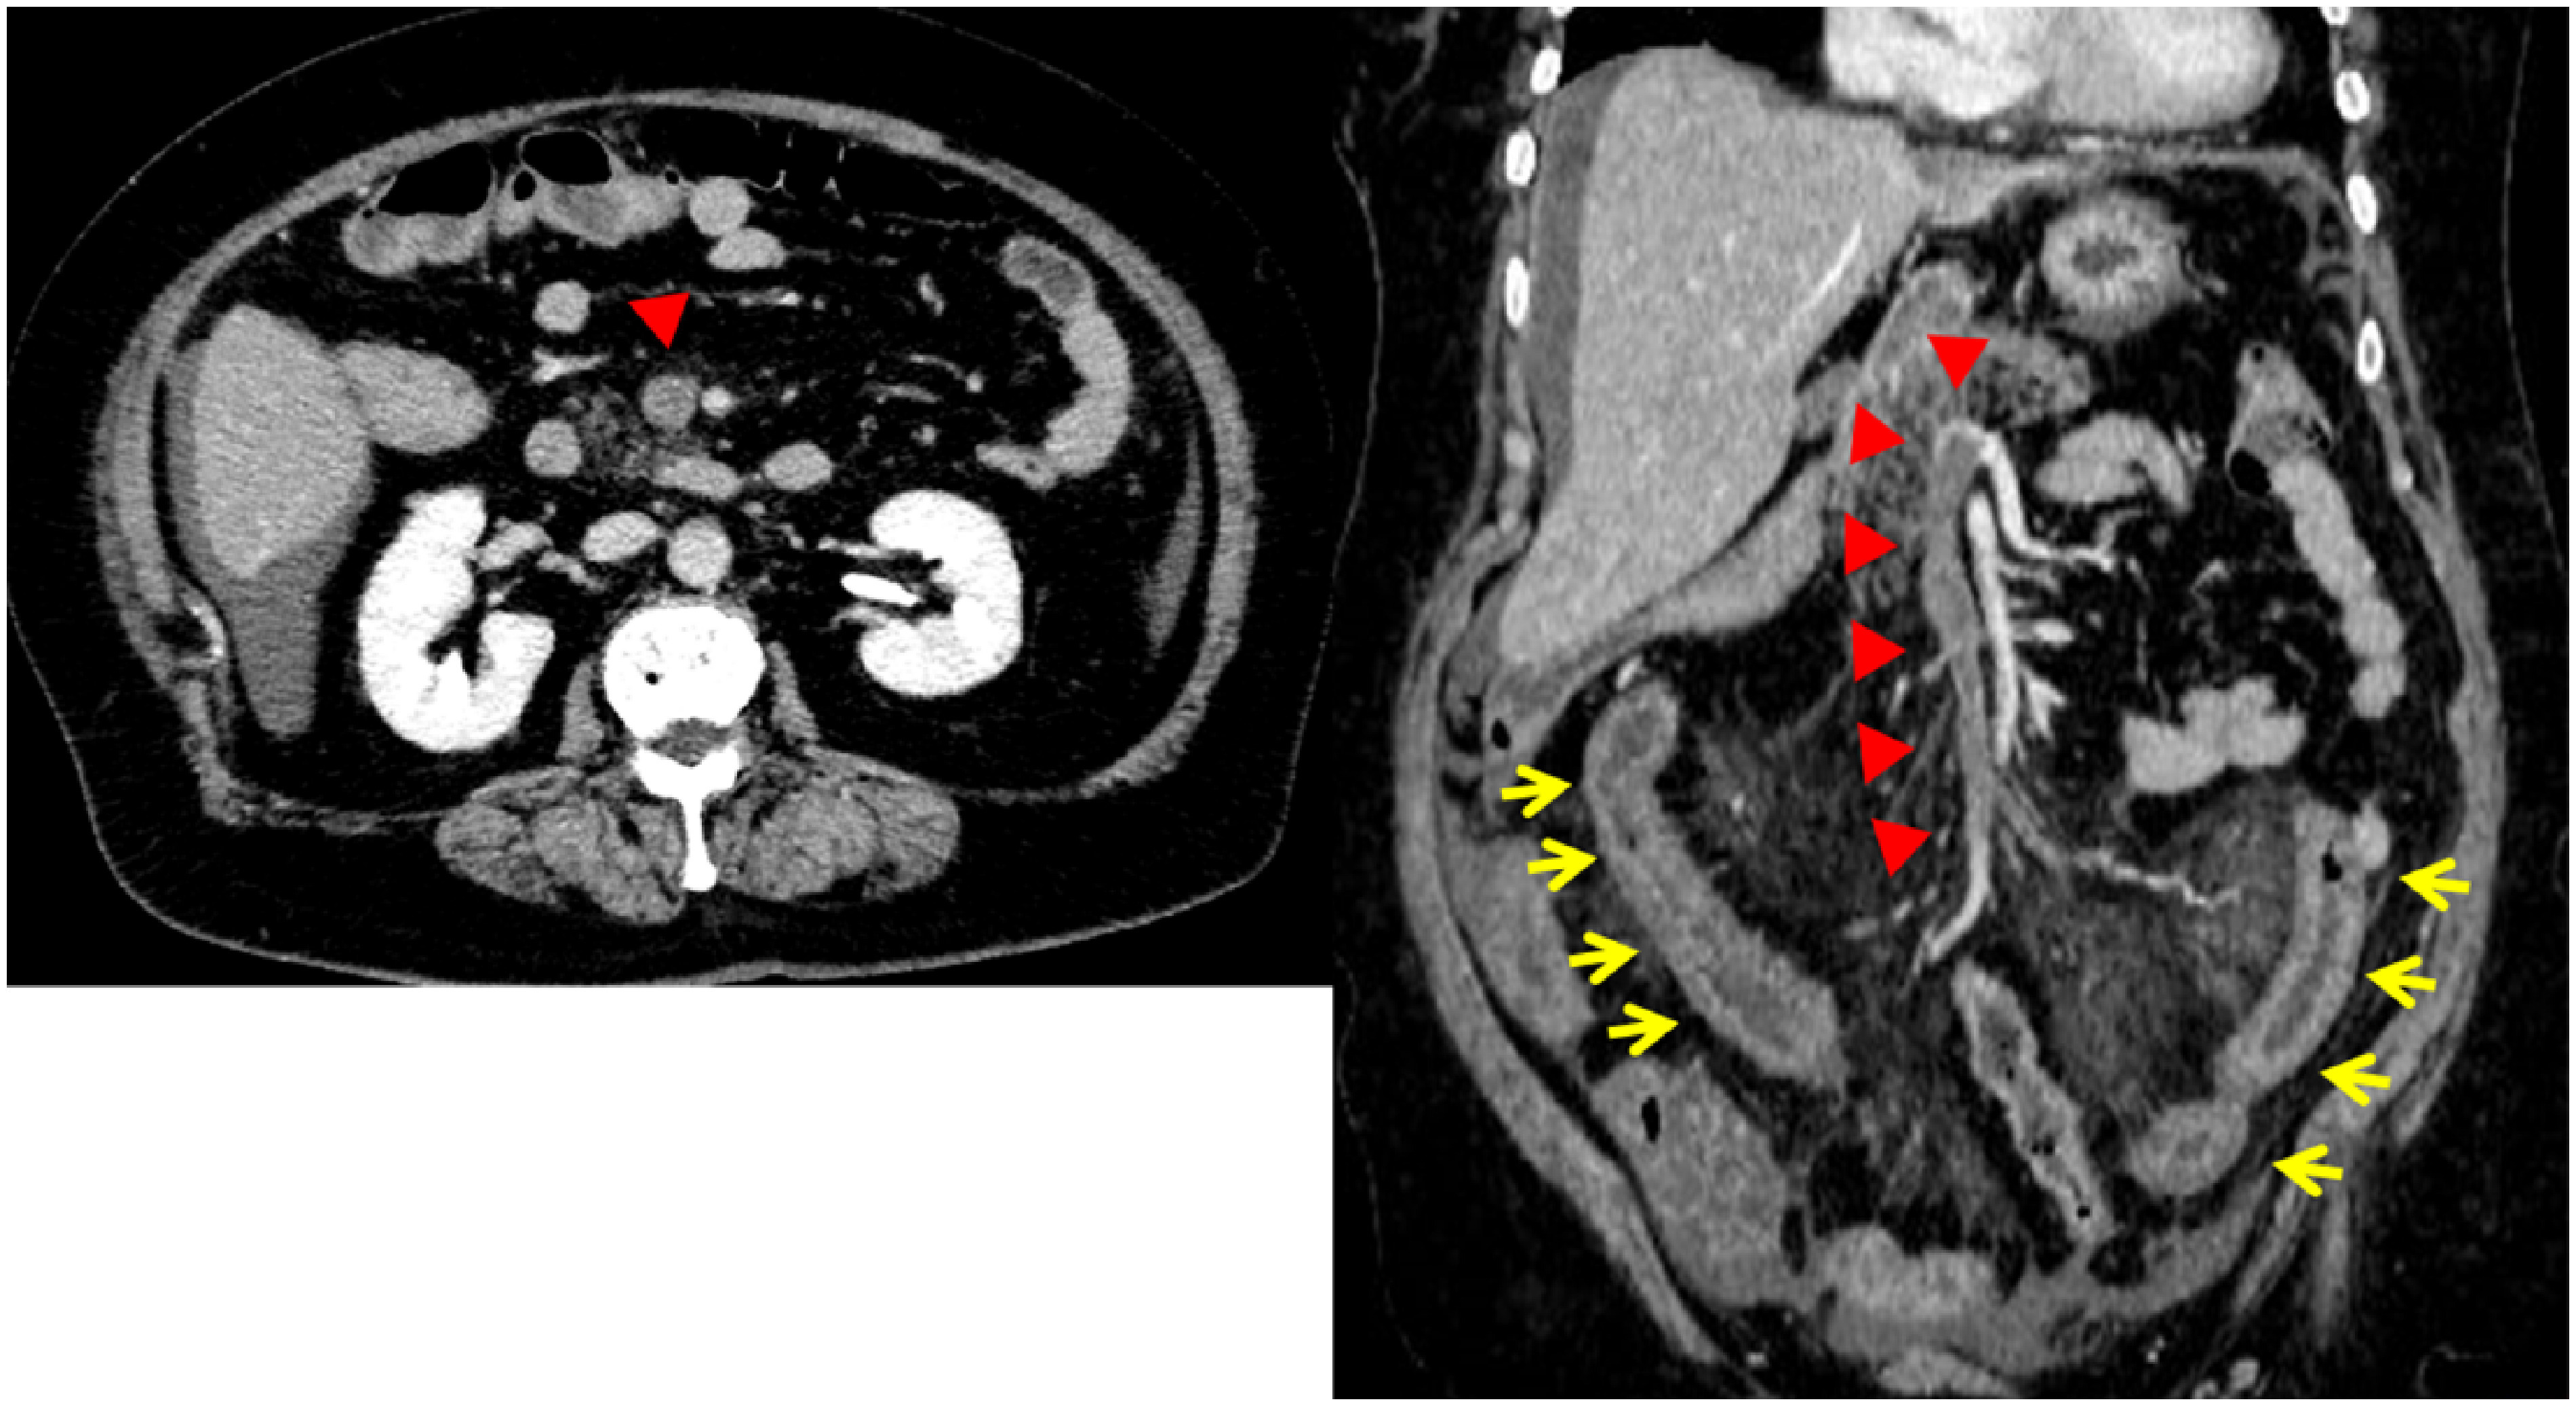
Fig. 1 Preoperative computed tomography of the abdomen and pelvis. A thrombus in the superior mesenteric vein extending to the splenic vein (arrowheads) is noticed along with edematous ileum and ascending colon (arrows).
